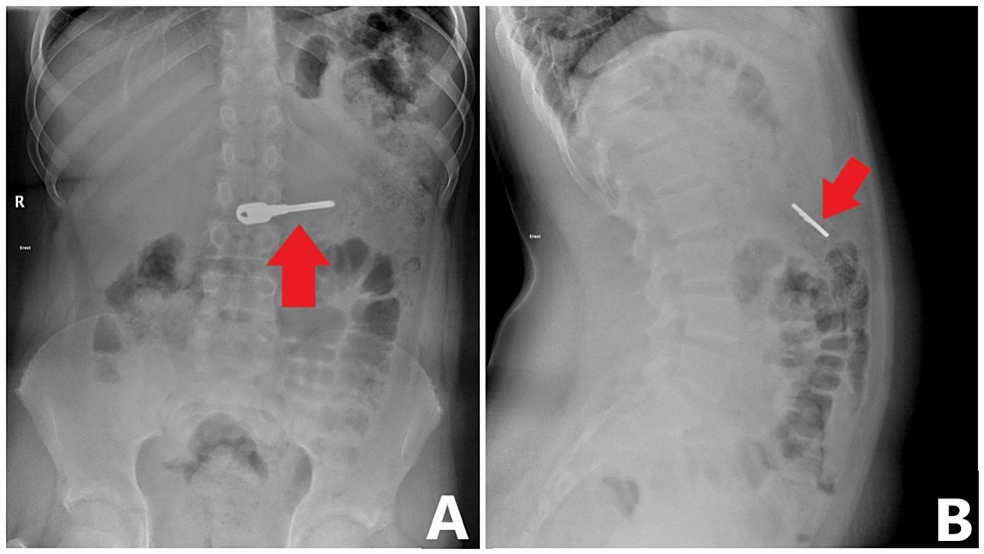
Erect abdominal X-rays reveal a radiopaque, metallic foreign body (red arrows)
